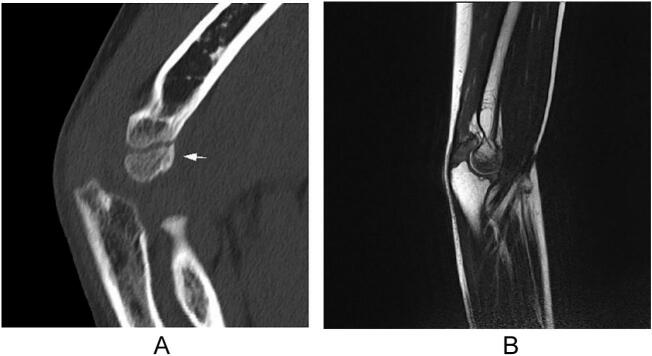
CT and MRI images at 4 months after surgery. (a) The arrow indicates the bone union site of the capitellum. (b) MRI shows a smooth articular congruity of the trochlea.
